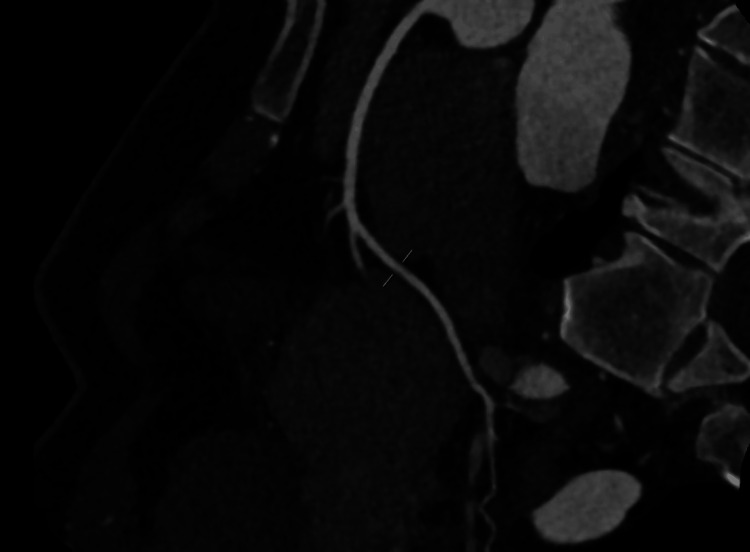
CT coronary angiogram showing patent right coronary artery
